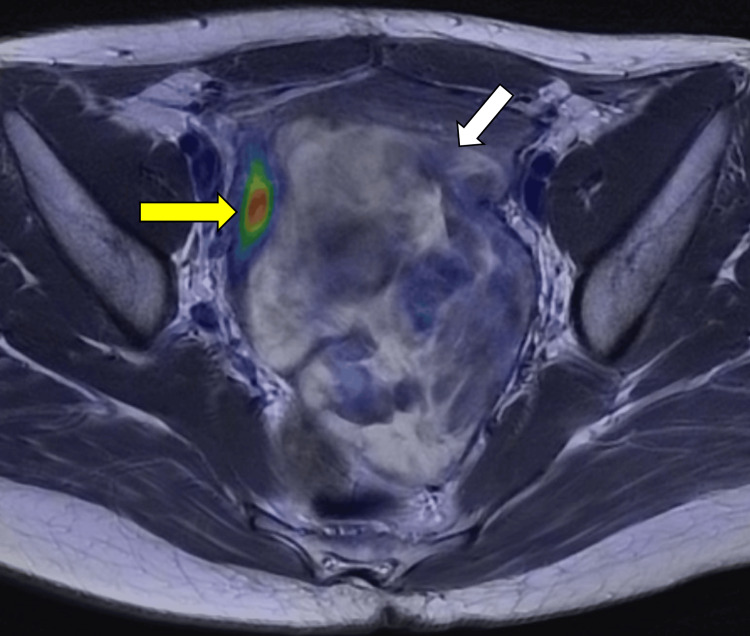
¹⁸F-fluoro-17β-estradiol (¹⁸F-FES) positron emission tomography (PET) findings ^18^F-FES PET MRI showed ^18^F-FES uptake was observed in the endometrium, and no evidence of accumulation in the tumor. (white arrow: tumor, yellow arrow: endometrium)
